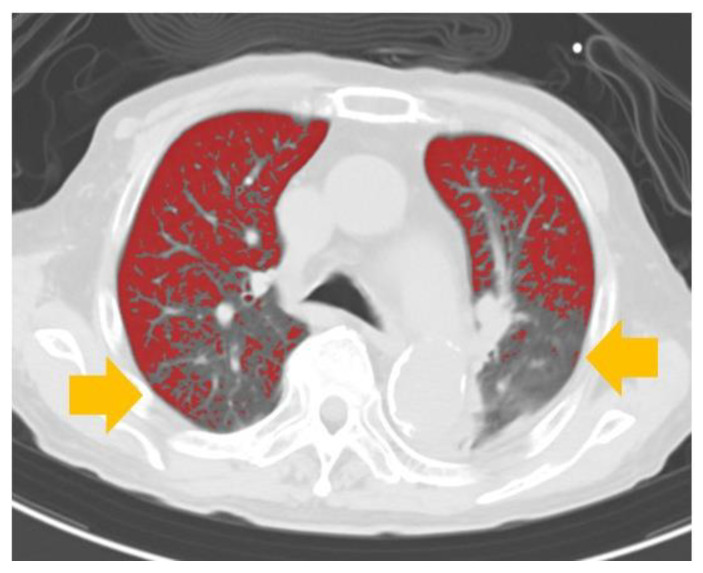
A representative case of pulmonary congestion. Chest CT image of a 75-year-old man with aortic stenosis and heart failure showing 34% ReDS and 56.9% high attenuation area. Yellow arrows in the bilateral lungs indicate hyperattenuated areas due to pulmonary congestion.
